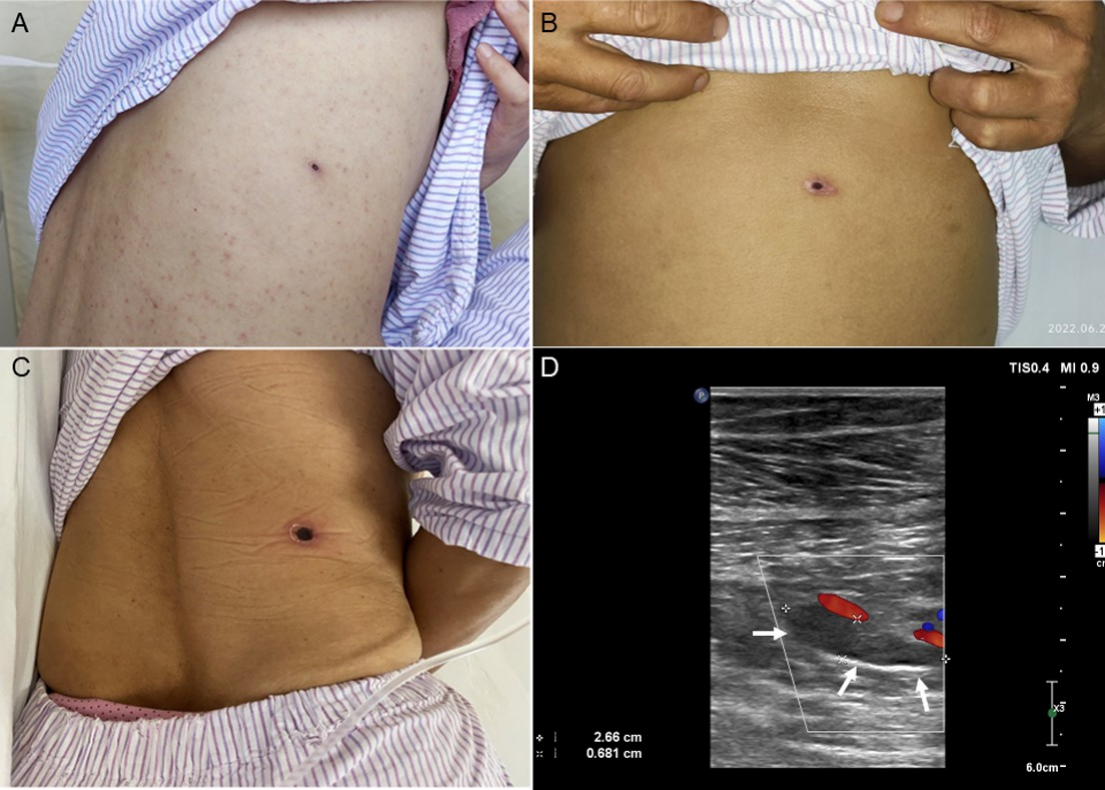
Clinical-radiological assessment of Case 4, 5 and 6. Panel A: eschar on lower back of Case 4; Panel B: eschar on abdominal wall of Case 5; Panel C: eschar on lower back of Case 6; Panel D: color doppler flow imaging for the left leg of Case 6, showing a 2.66 cm × 0.681 cm hypoechoic clot in the intermuscular vein lumen of the left leg (arrow), non-compressibility of the vein, and no spontaneous venous flow in the local intermuscular veins.
